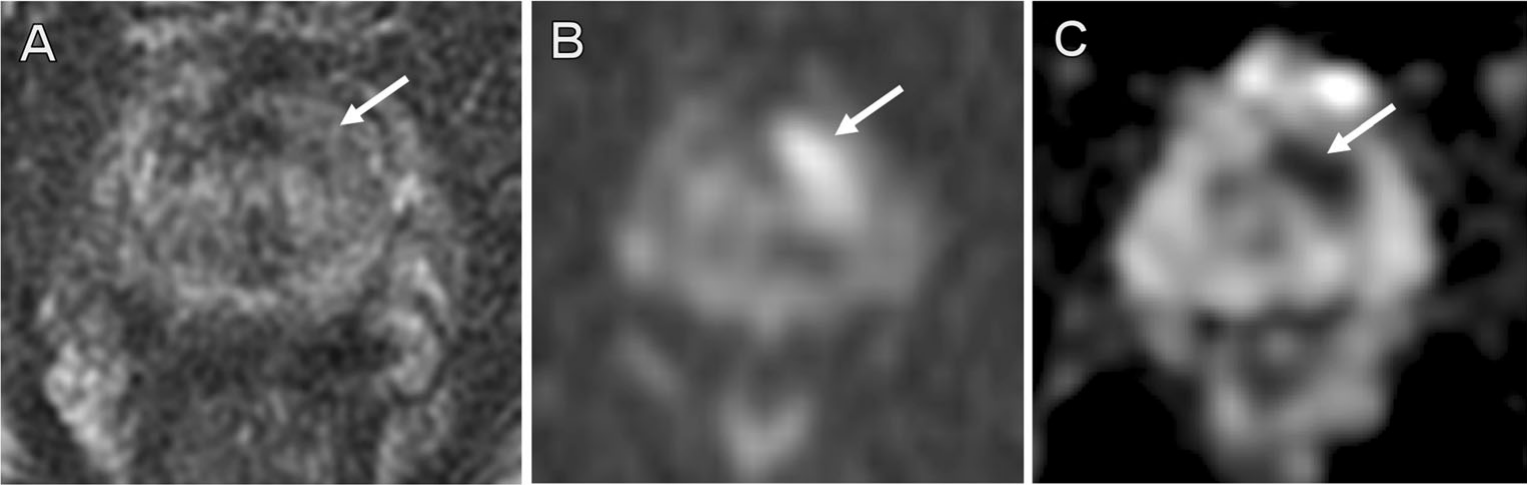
False-negative external report. A 70-year-old patient with 10.4 ng/ml PSA and previous negative TRUS biopsy. MRI reported as negative externally. Second report identified a high probability (PIRADS-5) 15-mm target in the left anterior apex transition zone (*arrows*), with homogeneous low T2-signal (a), and restricted diffusion on *b*-1,400 imaging (b) and ADC map (c). Transperineal biopsy found Gleason 3 + 3 disease in 30 % of both target cores
